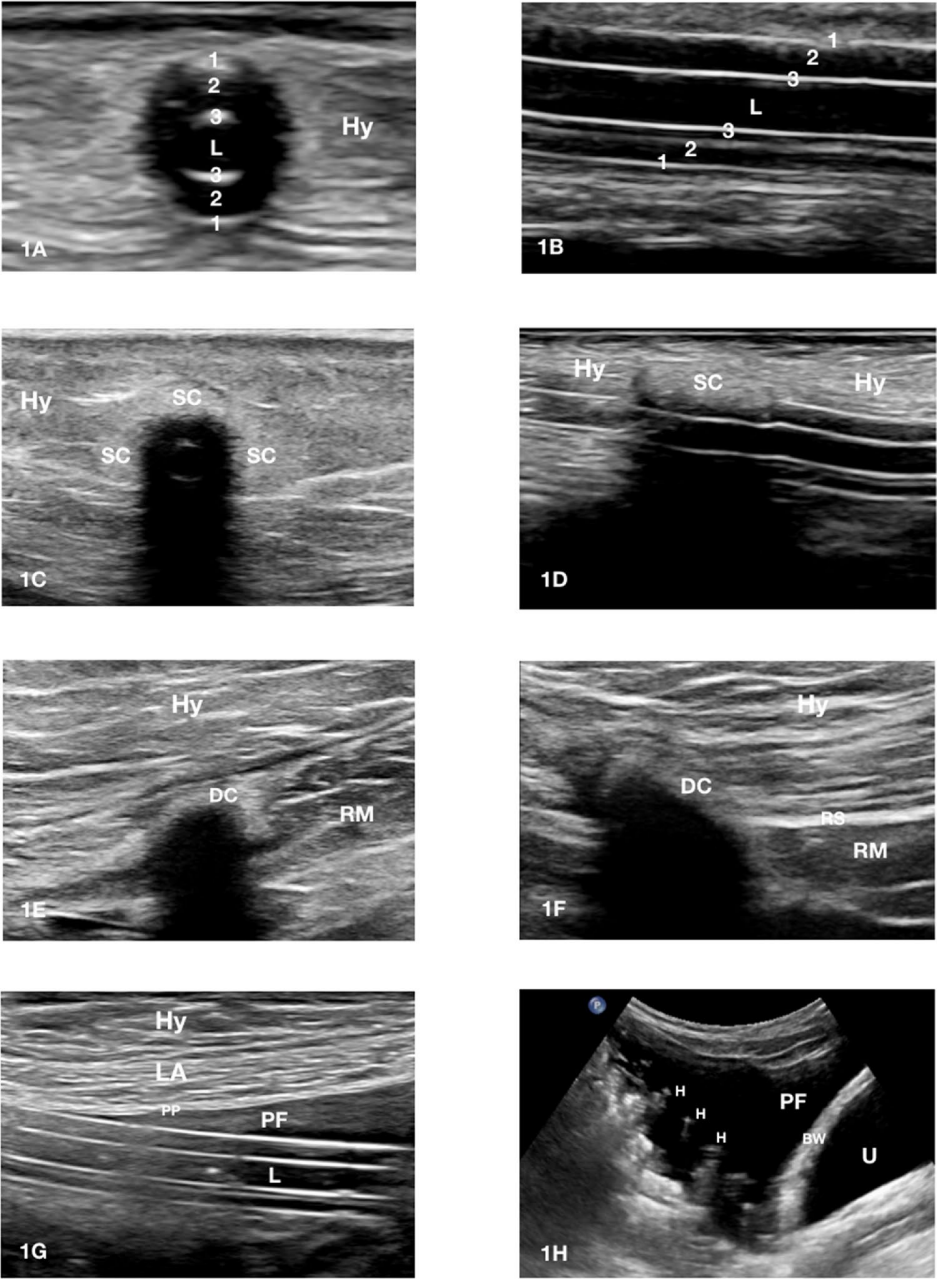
A–H normal ultrasonographic characteristics of the peritoneal catheter. A short-axis visualization of the catheter in the hypodermis (Hy) along the tract comprised between the exit-site and the superficial cuff. The catheter is visualized as a circular structure characterized by a trilaminar anterior wall (first thin hyperechoic rim [1], second hypoechoic layer [2], third hyperechoic rim [3]), an anechoic lumen (L) and a trilaminar posterior wall catheter (1,2,3); B long-axis visualization of the catheter in the subcutis along the tract comprised between the exit-site and the superficial cuff. The catheter is visualized as a “sandwich” structure characterized by a trilaminar anterior wall (1,2,3), an anechoic lumen (L) and a trilaminar posterior wall catheter (1,2,3); C short-axis visualization of the catheter at the level of the superficial cuff (SC). The cuff is visualized as a hyperechoic portion generating a posterior acoustic shadow; D long-axis visualization of the catheter at the level of the superficial cuff (SC). The cuff is visualized as a hyperechoic portion generating an acoustic posterior shadow cone; E short-axis visualization of the catheter at the level of the deep cuff (DC). The deep cuff possesses the same ultrasonographic features of the superficial cuff. In this case the deep cuff has been placed below the Hypodermis (Hy) within the fibers of the rectus abdominis muscle (RM). F long-axis visualization of the catheter at the level of the deep cuff (DC). In this case the deep cuff has been placed just above the rectus abdominis muscle (RM), (RS) = anterior rectus sheath; G long-axis visualization of the catheter at its passage in the peritoneal cavity. In this case the peritoneal catheter has been inserted in midline position and the deep cuff placed at the level of the linea alba (LA), as described elsewhere [48]. PP = parietal peritoneum; PL = peritoneal fluid; L = catheter lumen; H Visualization via convex probe (frequency range 2.5–6 MHz) of the intra-peritoneal tract of the catheter. The device is identified in the peritoneal fluid (PF) as a slightly bent curve made up of several hyperechoic spots generated by the lateral holes (H) on its distal part. In this case the tip of the catheter leans against the posterior wall of the bladder (BW) in Douglas’ pouch, U = urine
